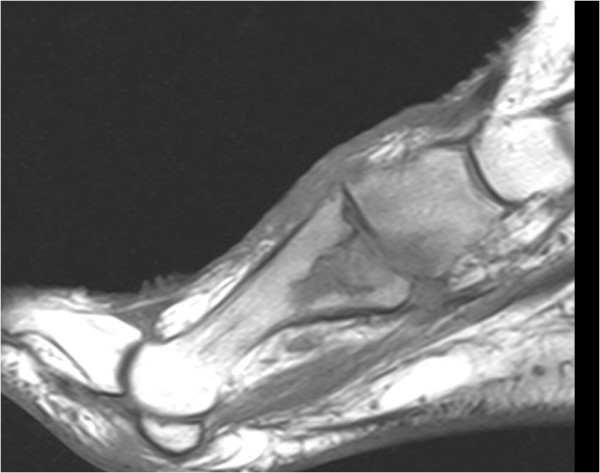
A magnetic resonance imaging scan of the foot showing the destructive changes of osteomyelitis in the proximal first metatarsal bone and adjacent medial cuneiform bone.
